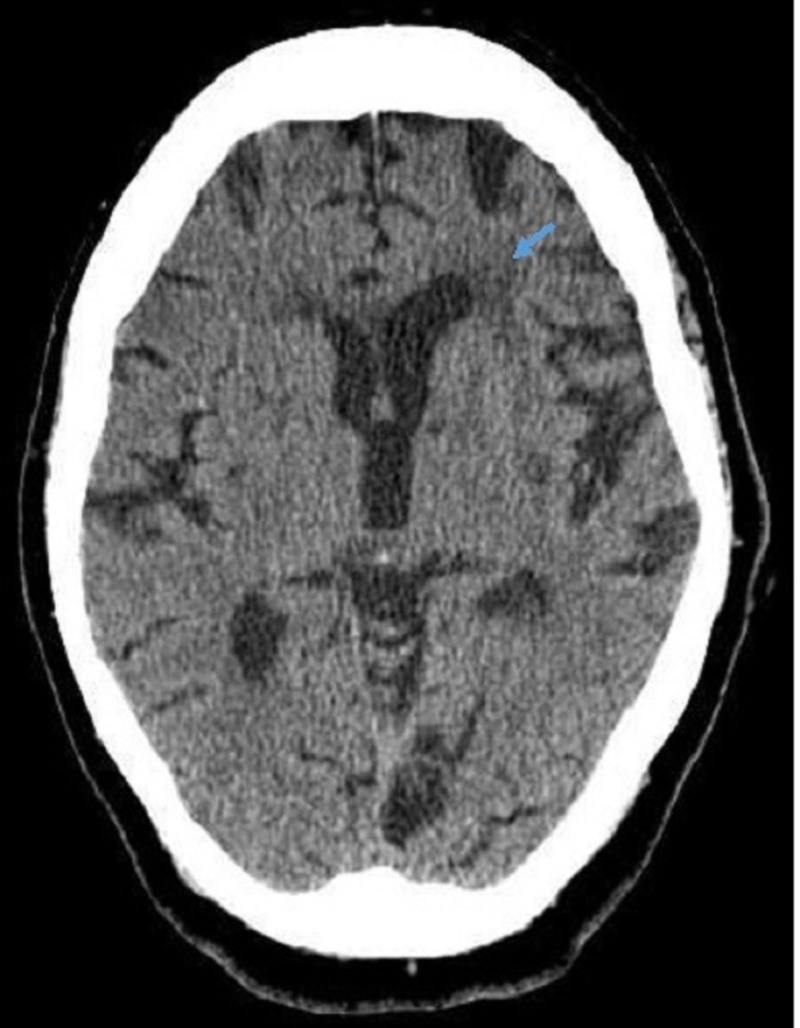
CT head showed an old infarct (blue arrow)
